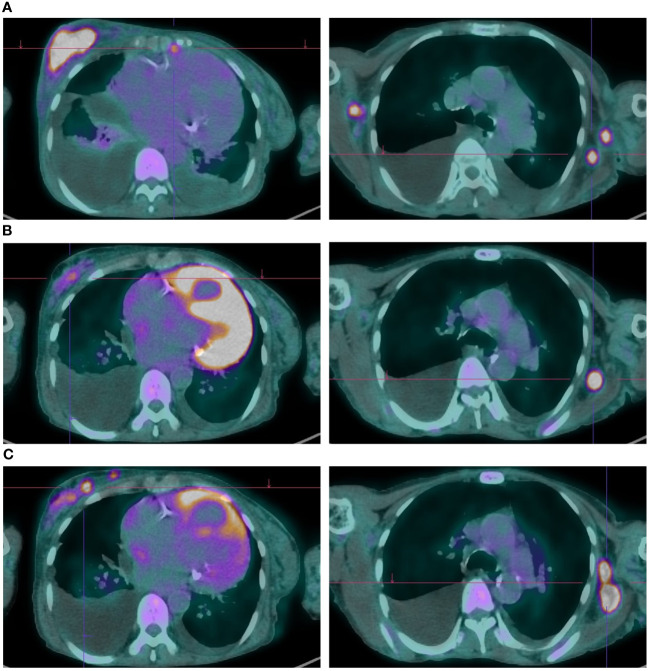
Metabolic and radiologic evaluation on FDG-PET of cancer lesions throughout Phesgo® treatment. (A) Obtained on July 22, 2022; showed disease progression with an increased atypical lesion in the right breast and bilateral lymphadenopathies. (B) Captured on January 10, 2023; demonstrated a favorable response to Phesgo® treatment with reduced breast lesion size and improved lymphadenopathies. (C) Obtained on April 20, 2023; indicated disease progression with new hypermetabolic foci, pleural effusion, ascites, and lytic lesions.
